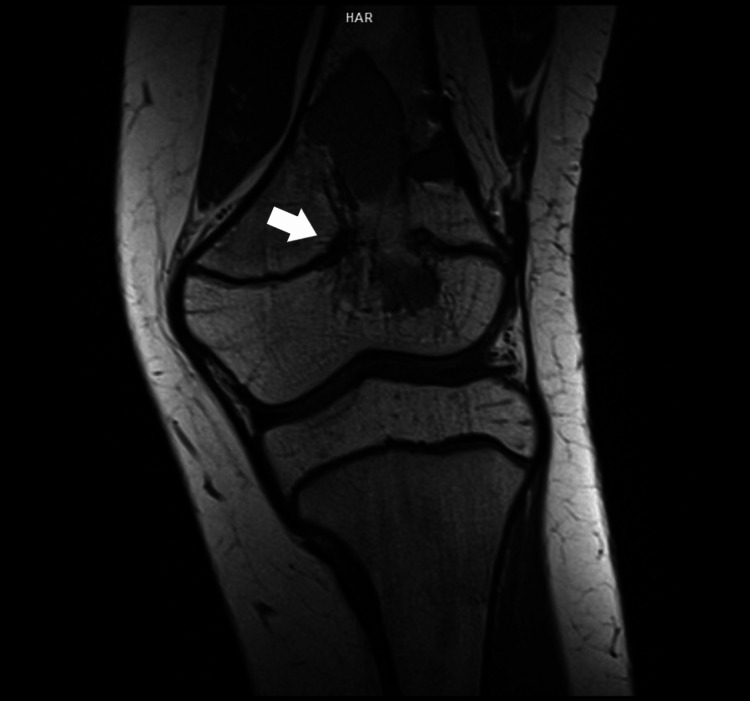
Coronal T1 MRI image displays the diminished diameter of the remaining cyst elements, alongside disturbance of the normal growth plate line.
